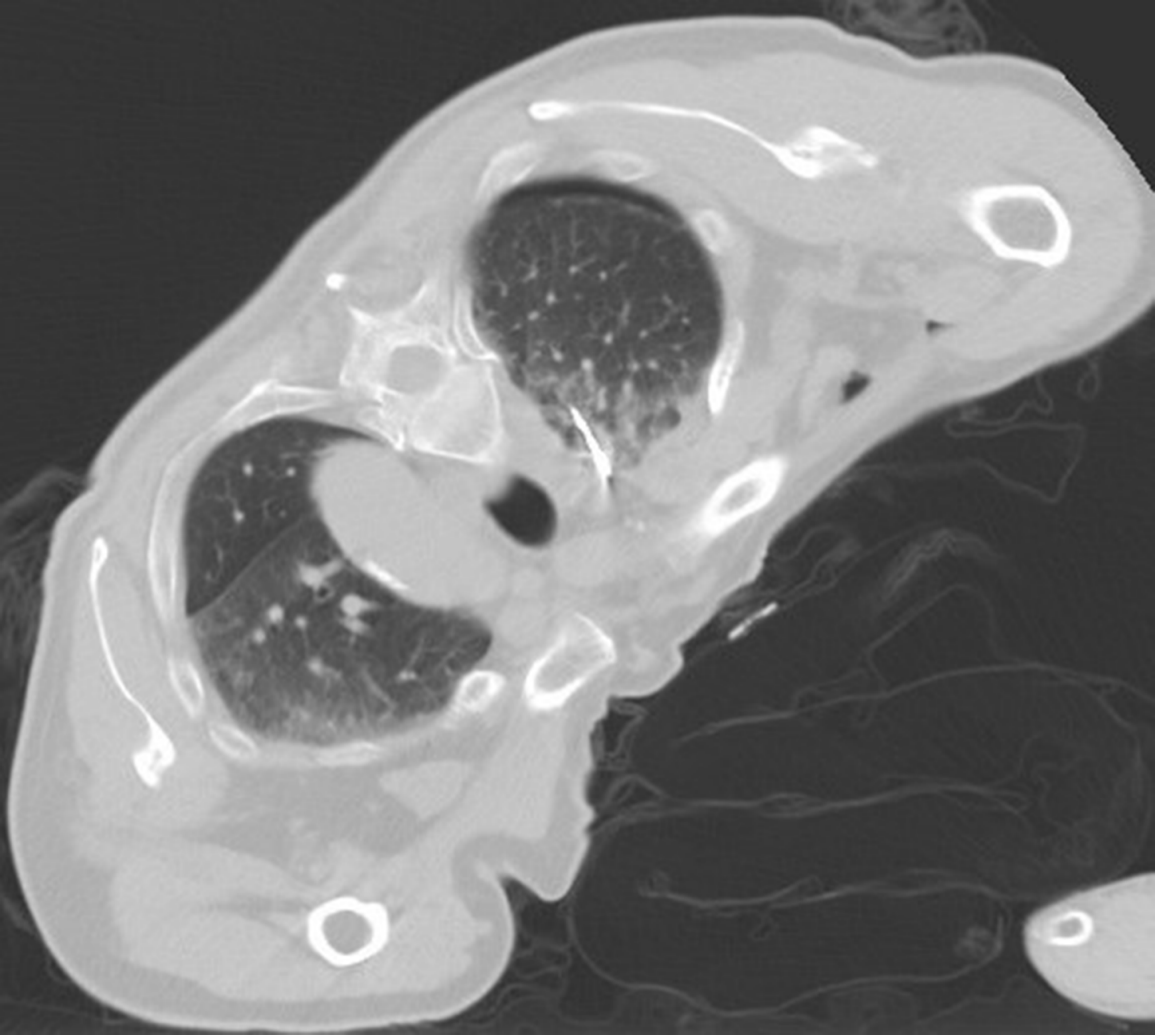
Guided by CT, the needle was gradually inserted through the microwave antenna to the bottom of the lesion for ablation.
